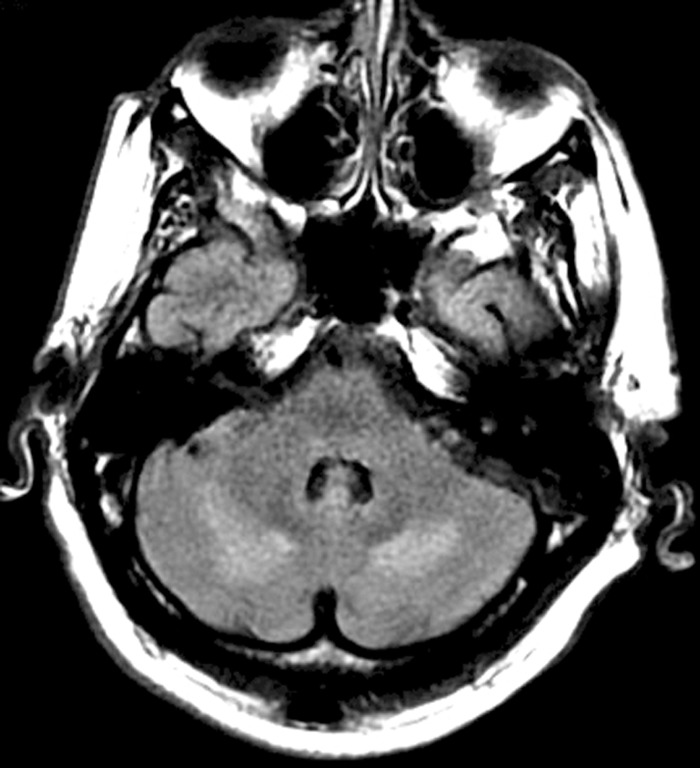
Initial fluid attenuated inversion recovery (FLAIR) MR sequence demonstrating hyperintense lesions adjacent to the cerebellar dentate nuclei bilaterally. No additional lesions were identified on this MR series.
